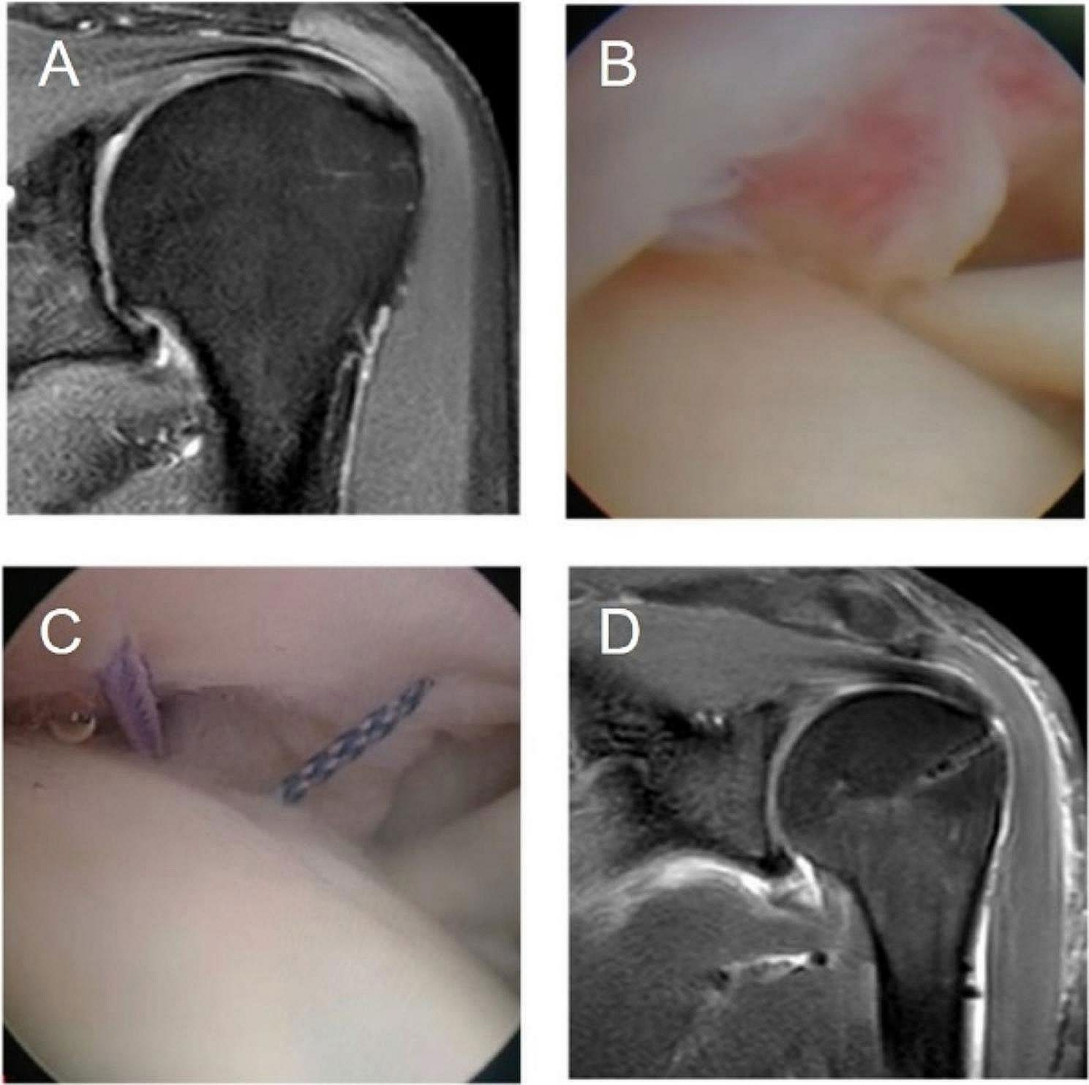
The patient underwent the arthroscopic trans-tendon repair method (A) Preoperative magnetic resonance imaging showed an Ellman III partial articular-sided supraspinatus tendon tear; (B) Partial tear of the supraspinatus tendon at the articular side in the glenohumeral joint; (C) Sutures were introduced into the glenohumeral joint; (D) Postoperative magnetic resonance imaging indicated satisfactory repair of the rotator cuff
